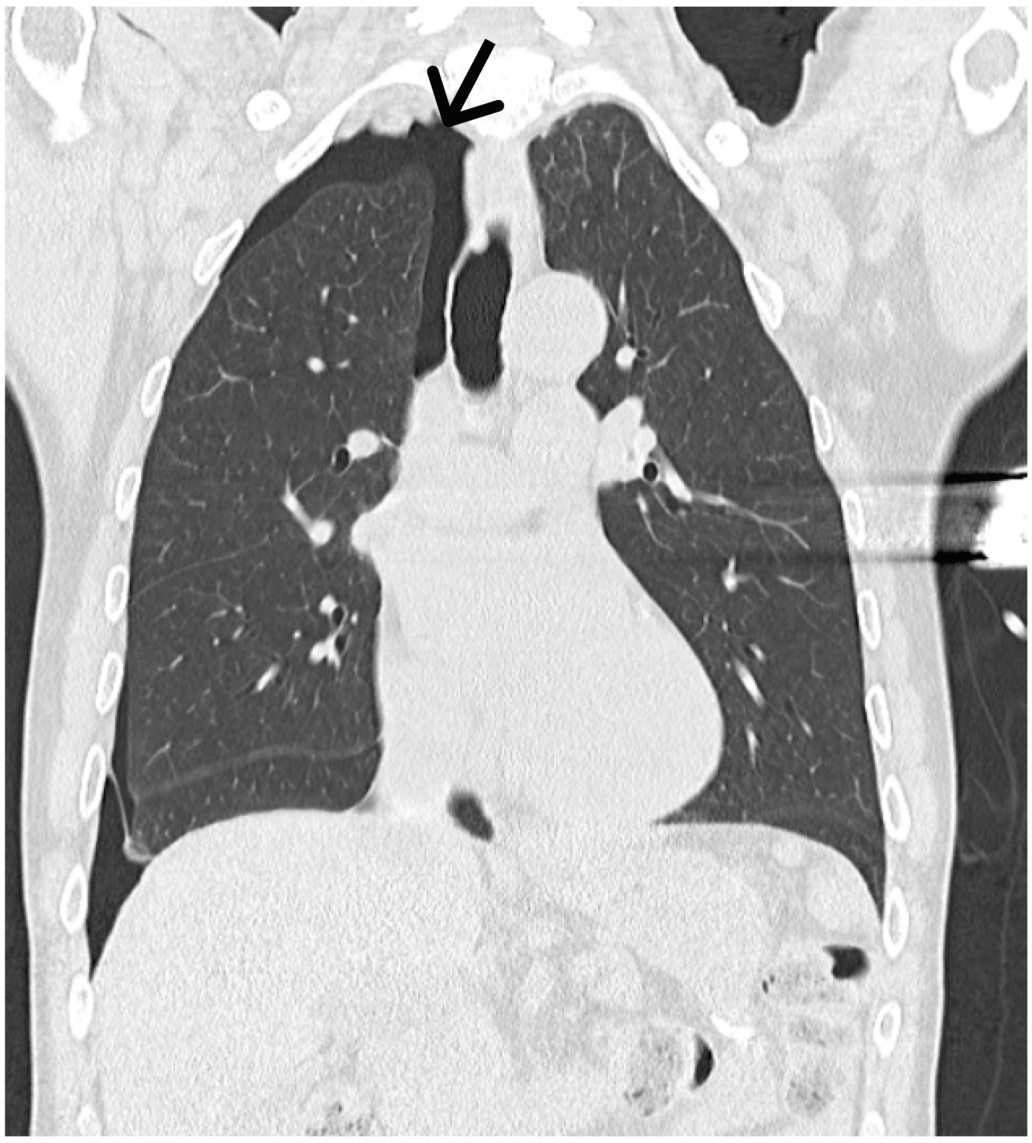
Computerized tomography (CT) scan of the chest evidencing the iatrogenic pneumothorax (arrow) on the patient’s right side in a coronal axis cut.
